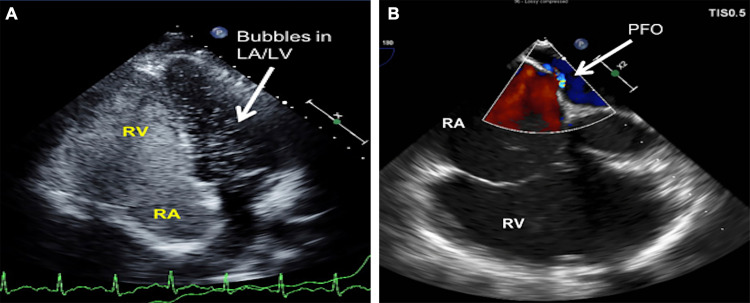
(A) Four-chamber TTE image with agitated saline contrast study demonstrating microbubbles in the LV suggestive of a PFO (arrow). (B) Right-sided focused, intraoperative color Doppler TEE image demonstrating PFO with right-to-left shunt (arrow). LA, left atrium; LV, left ventricle; PFO, patent foramen ovale;RA, right atrium; RV, right ventricle; TEE, transesophageal echocardiogram; TTE, transthoracic echocardiogram
